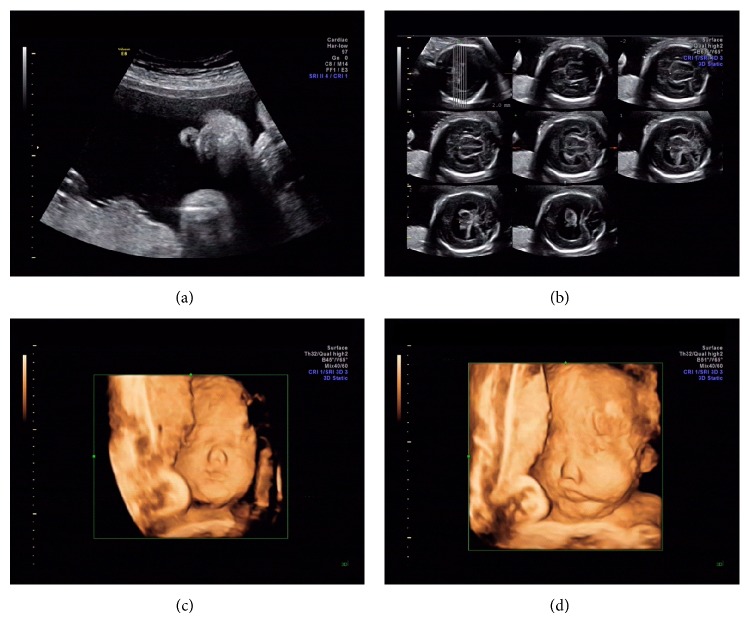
Sonographic findings in Case 1 at 23^+1^ weeks. (a) In two-dimensional coronal plane, single nostril was visualized. (b) Holoprosencephaly was demonstrated in tomographic ultrasound imaging (TUI). ((c) and (d)) Single nostril was further confirmed in three-dimensional imaging.
